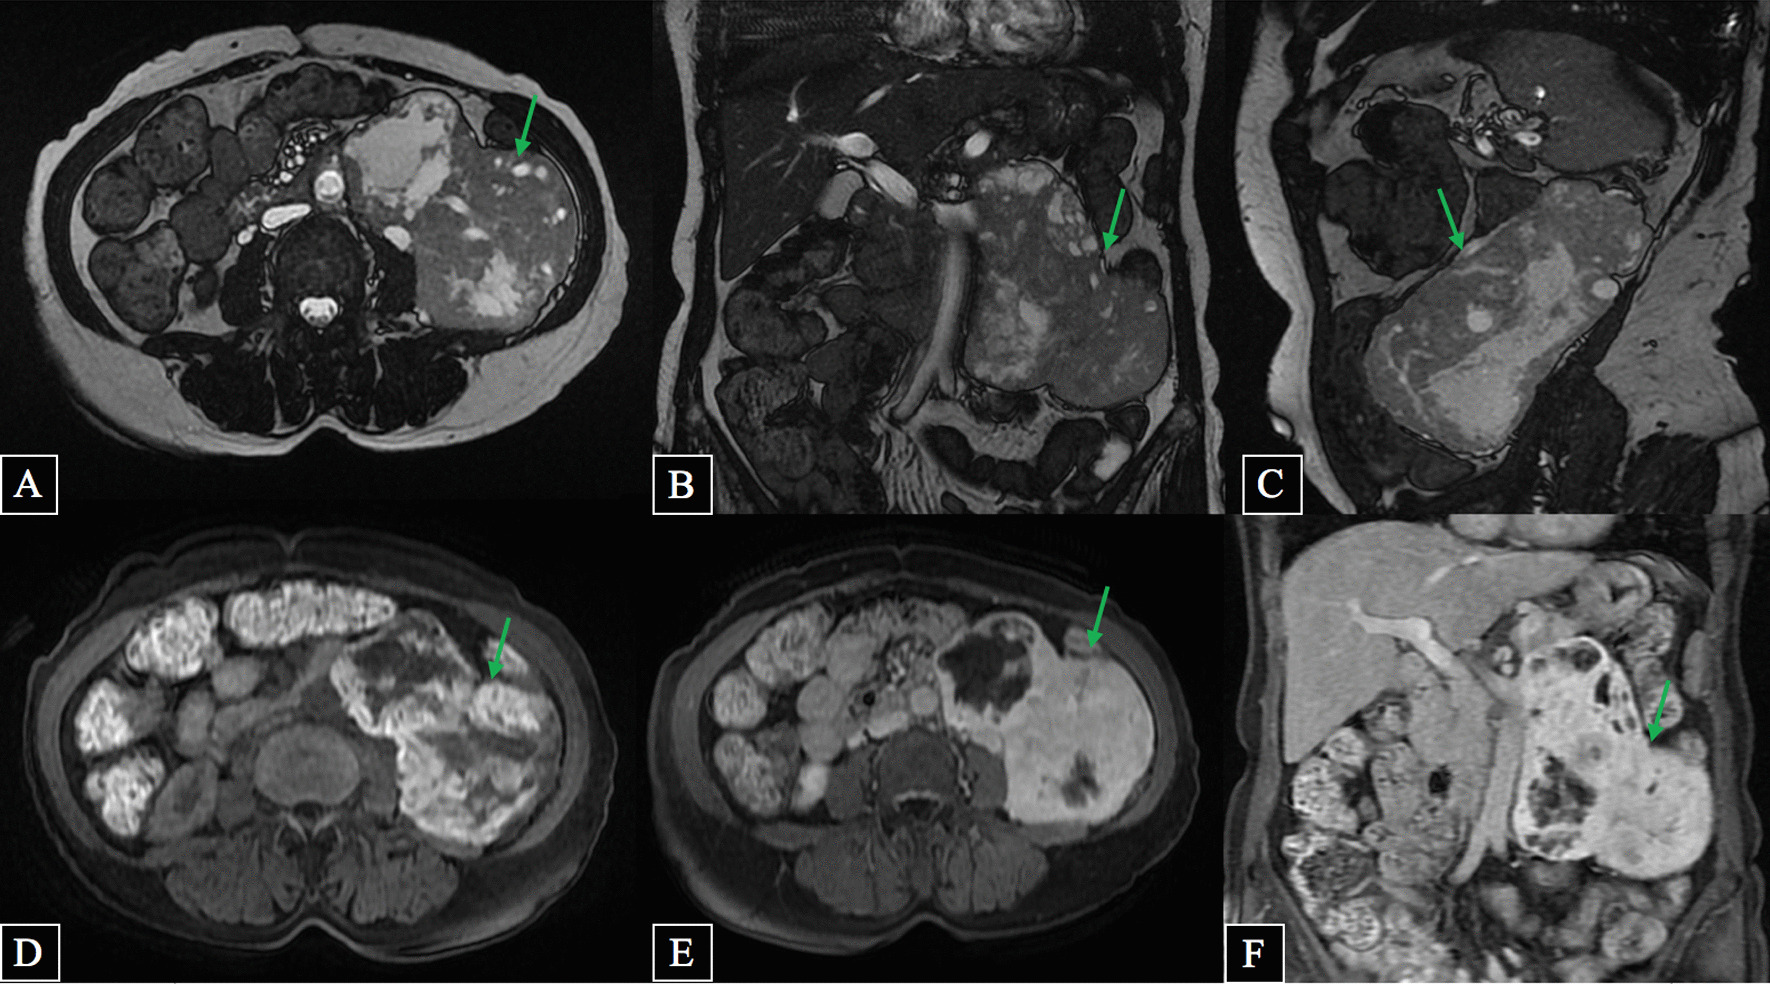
MRI in axial, coronal, and sagittal T2w (A–C), axial T1w (D), and axial and coronal T1 Gd (E, F) showing large retroperitoneal paraganglioma (
